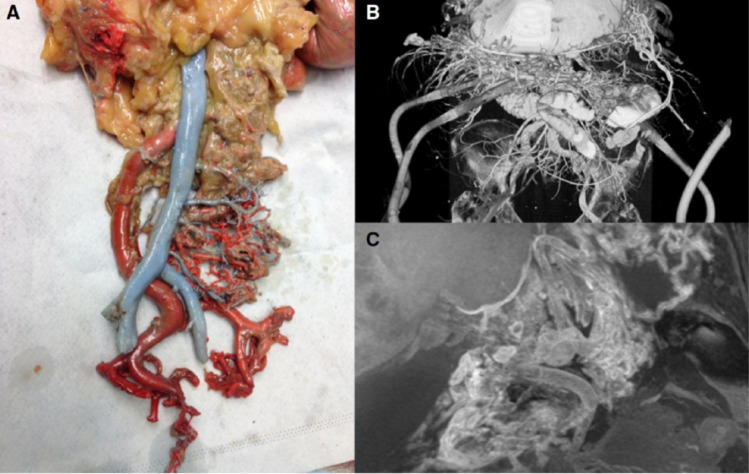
Dissection of the vascular anatomy of the human pancreas following injection of a contrast-enhanced mixture (latex-gadobutrol-barite). a Gross anatomical view of the vascular network with arteries labeled in blue and veins in red. b Corresponding CT image showing high-resolution visualization of the injected vessels. c MRI image of the same region, demonstrating complementary soft tissue contrast and vascular delineation. Adapted from Renard et al. [30]; © 2024 Anatomical Society / Wiley. Reproduced with permission
